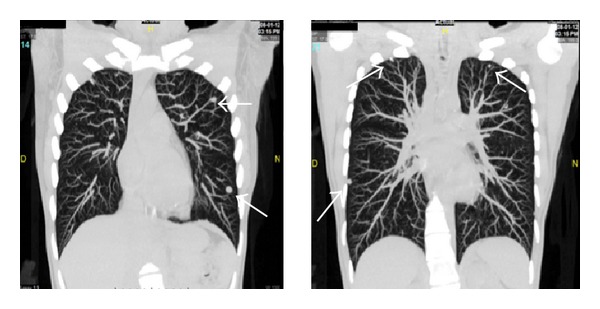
Pulmonary nodules (arrows) in both hemithoraces on a CT scan.
